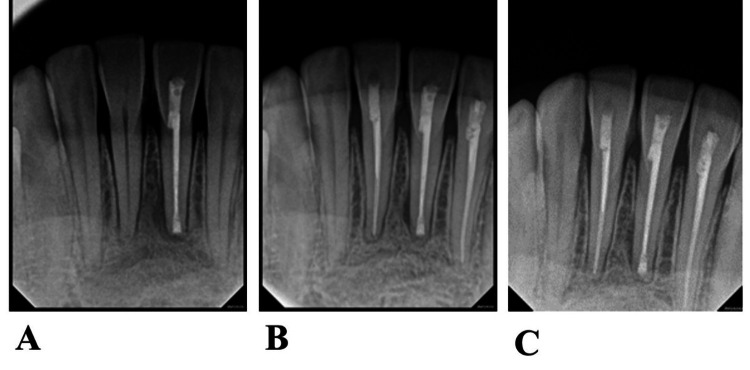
(A) Periapical radiograph after 12, (B) 24 and (C) 36 months’ follow-up A subsequent examination was conducted after 12, 24, and 36 months (follow-up) (Figure 4A-4C), which revealed a decrease in the size of the lesion. Additionally, no pain was reported during percussion and palpation. Furthermore, there was no observation of vestibular swelling.
